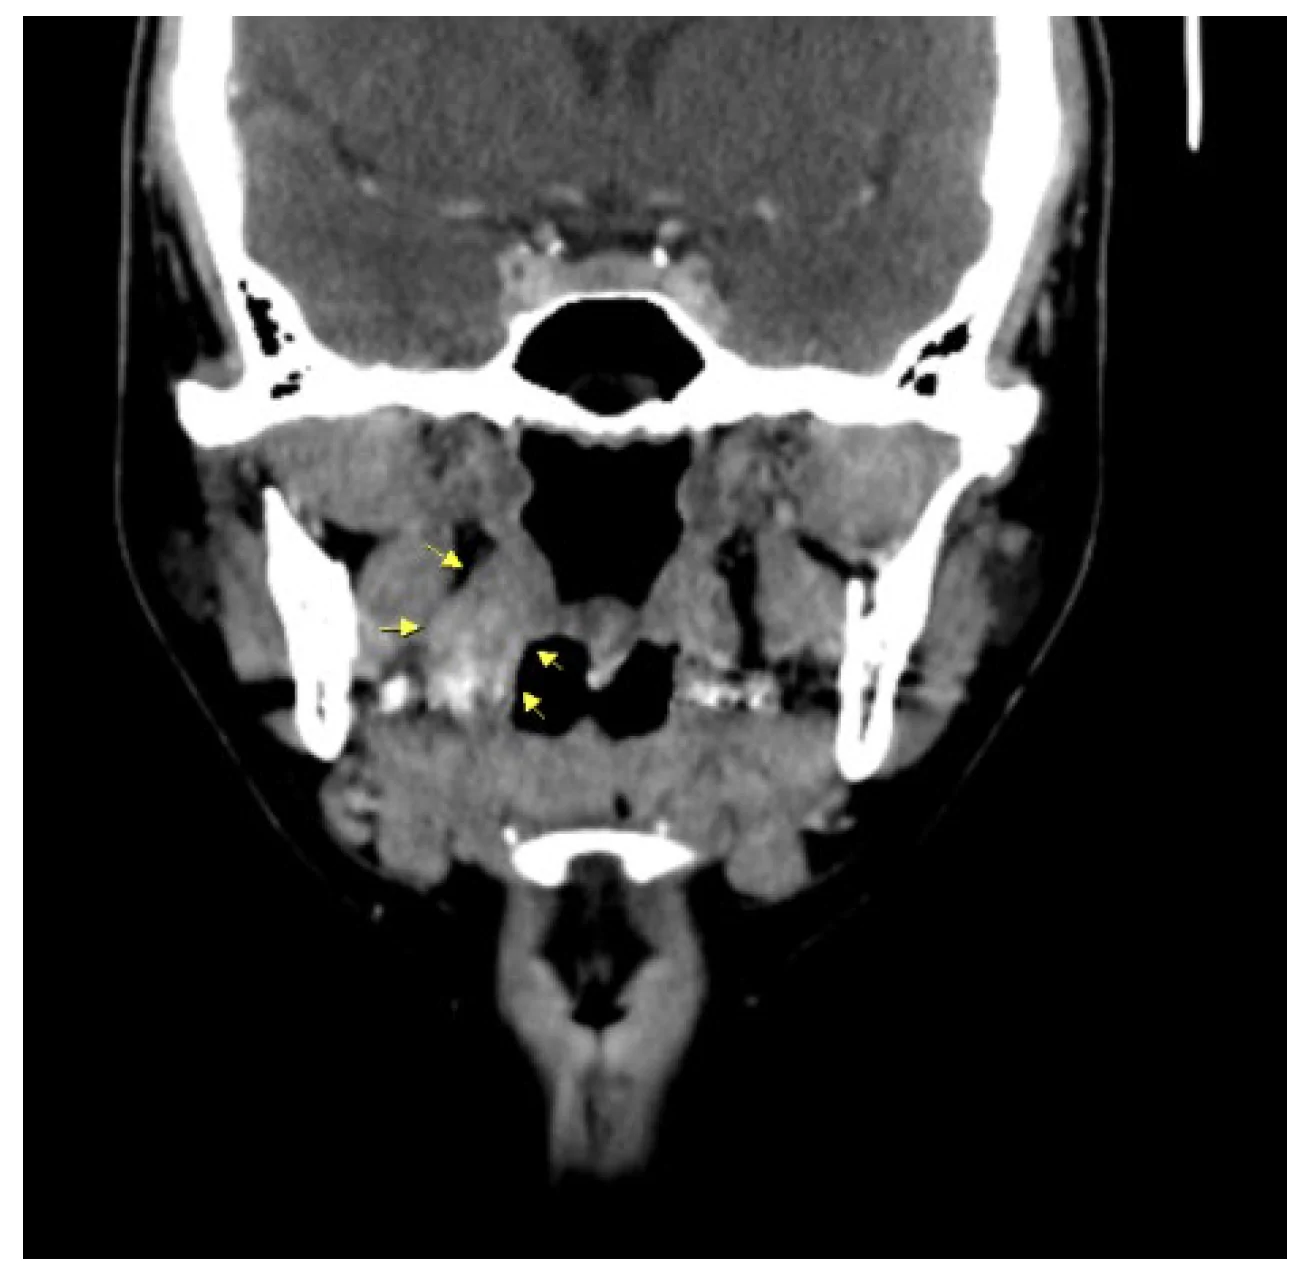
Contrast-enhanced CT showing asymmetric enlargement of the right palatine tonsil with effacement of the adjacent parapharyngeal fat plane, suggestive of a submucosal lesion.
